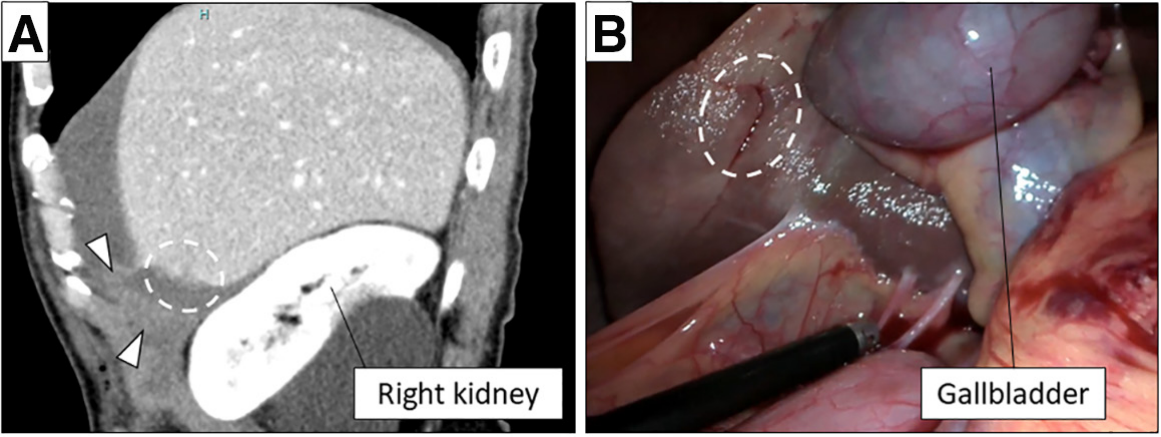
Retrospective review of preoperative CT images and operative video. (A, B) This review revealed that a large amount of hematoma had accumulated in the right subphrenic space (arrow head), and a small laceration was present on the surface of liver segment 5 (dashed-line oval).
